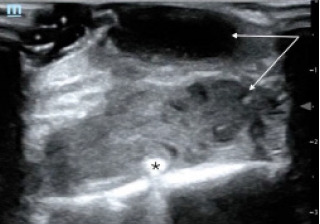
A point-of-care ultrasound view of the anterior chest wall, showing a complex fluid collection with irregular borders and mixed echogenicity (arrows) and surrounding inflammatory changes extending deep to the hyperechoic driveline (star).
